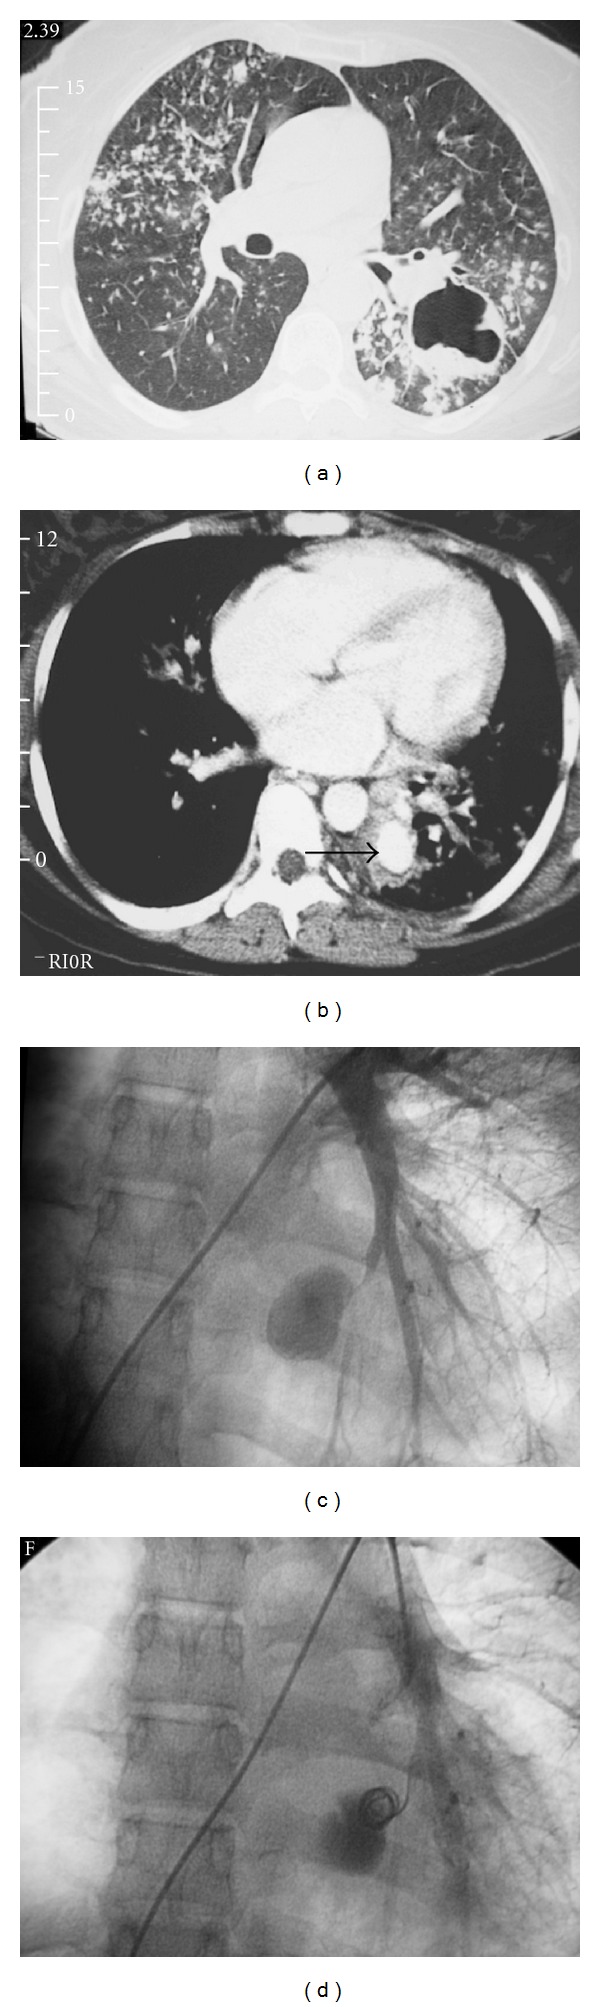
(a) Lung CT demonstrates bilateral nodular infiltrates with a left lower lobe cavity containing hematoma from active pulmonary tuberculosis. (b) Contrast CT demonstrates focal contrast enhancement in the left lower lobe pulmonary aneurysm (arrow). (c) Selective left lower pulmonary arteriogram demonstrates the saccular Rasmussen aneurysm with a narrow neck. (d) Post embolization pulmonary angiogram demonstrates occlusion of the neck of the aneurysm with a single steel coil with residual contrast within the aneurysm.
